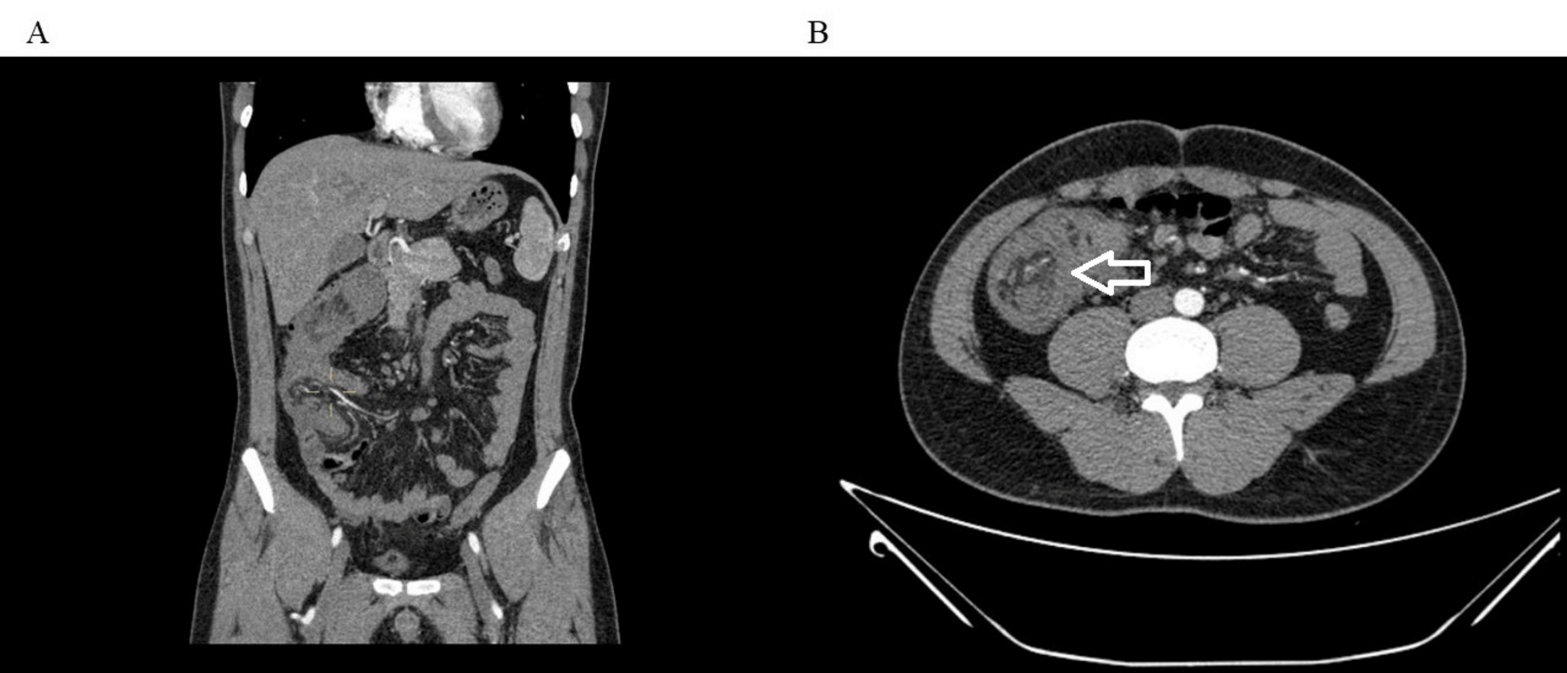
Enhanced abdominal CT imaging. (A) Coronal plane showing the terminal ileum and mesentery entering the cecum (yellow cross). (B) Axial plane demonstrating the "target sign" CT: Computed tomography
